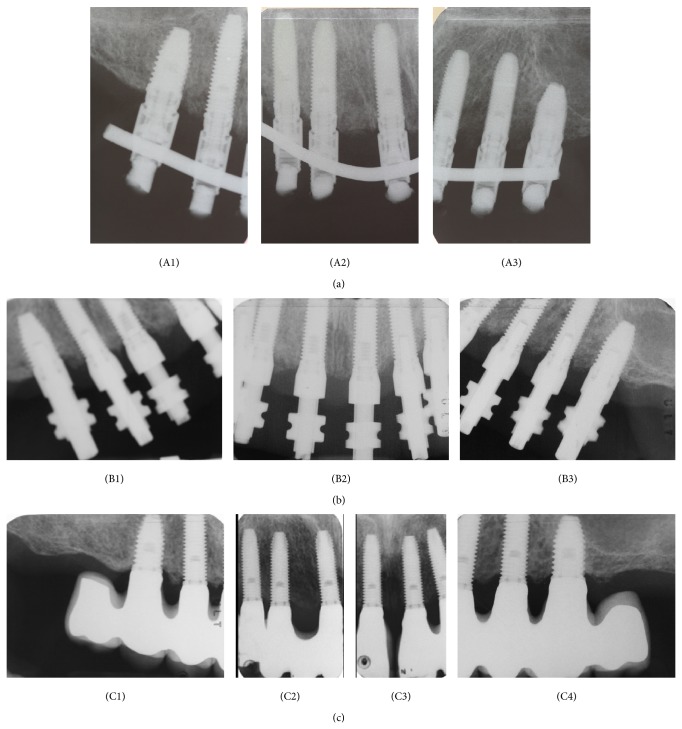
Radiographic examination: (A1), (A2), and (A3): postoperative radiographs. (B1), (B2), and (B3): at 6 months during the impression for the final restoration. (C1), (C2), (C3), and (C4): 18 months after the surgery.
